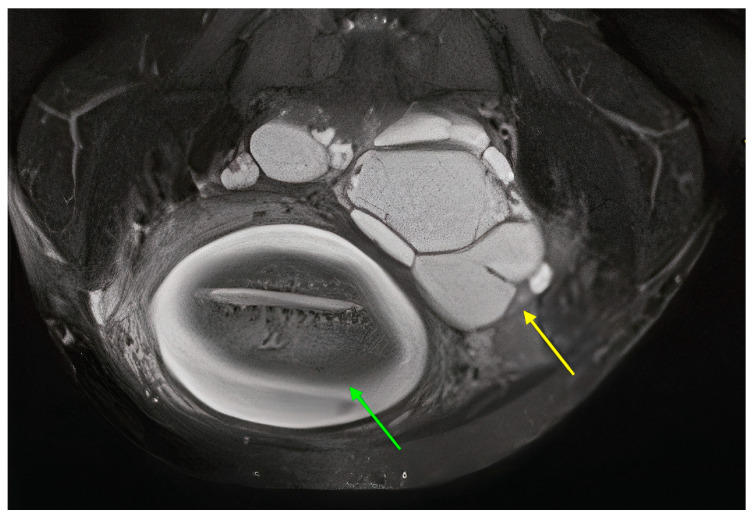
Abdominal MRI, transverse view of the fetus (green arrow) and a high-grade serous ovarian cancer (yellow arrow).
